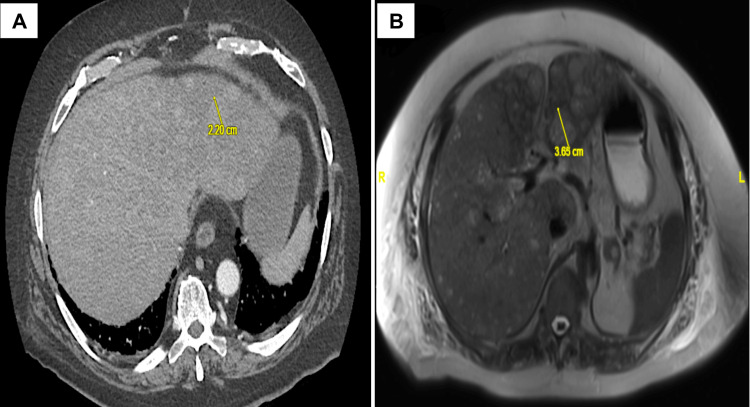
A: Transverse CT abdomen demonstrating a lesion in the left hepatic lobe. B: Follow-up MRI with diffuse lesions throughout the liver.
